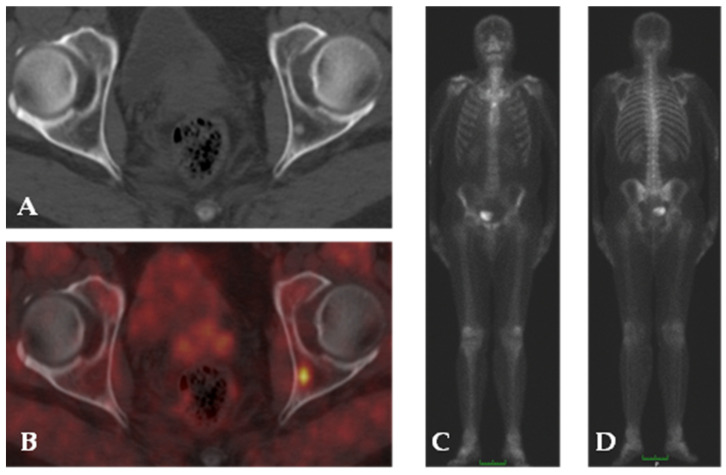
^68^Ga-PSMA PET/CT imaging in a patient with PC. Co-registered CT (A) shows hyper-dense area in the left iliac bone with no evidence of focal uptake of ^68^Ga-PSMA on fused images (B). ^99m^Tc-MDP bone scan anterior and posterior projection (C,D) shows normal tracer distribution.
